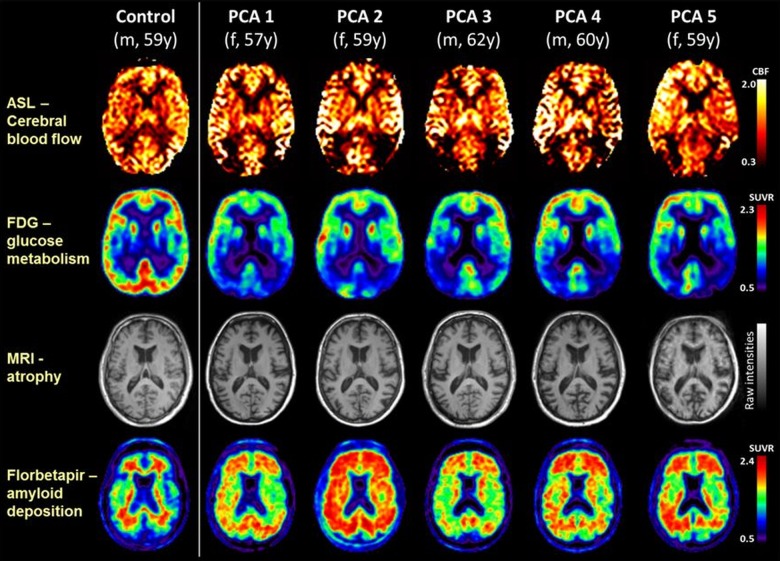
Single-participant axial images for one control participant and five patients with PCA showing cerebral blood flow (ASL), glucose metabolism (FDG-PET), atrophy (structural MRI), and amyloid deposition (florbetapir-PET). For clinical purposes, 18F-florbetapir images should be read on a grey scale. ASL, arterial spin labelling; CBF, cerebral blood flow; FDG-PET,^18^ F-labelled fluorodeoxyglucose positron emission tomography; PCA, posterior cortical atrophy; SUVR, standard uptake value ratio.
